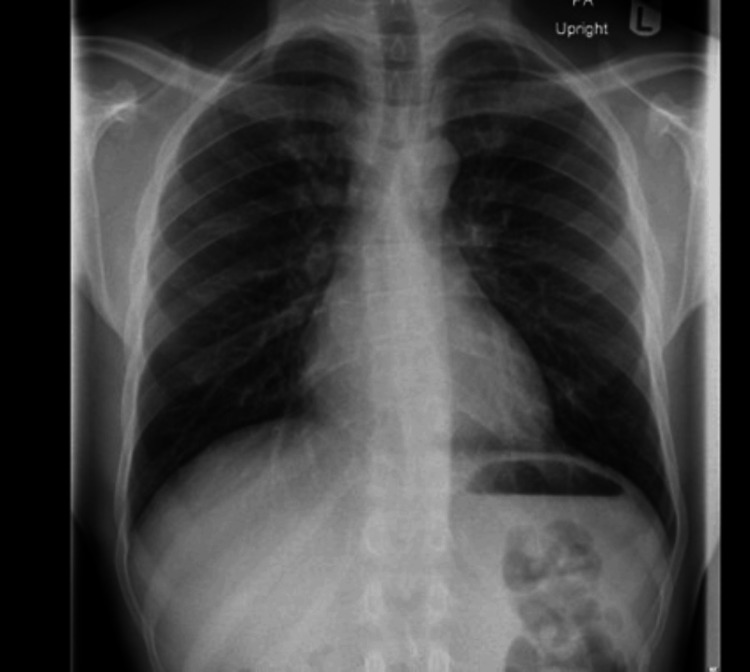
Chest X-ray (PA view) CXR revealed improvement in both pleural and pericardial effusion. PA: posteroanterior
